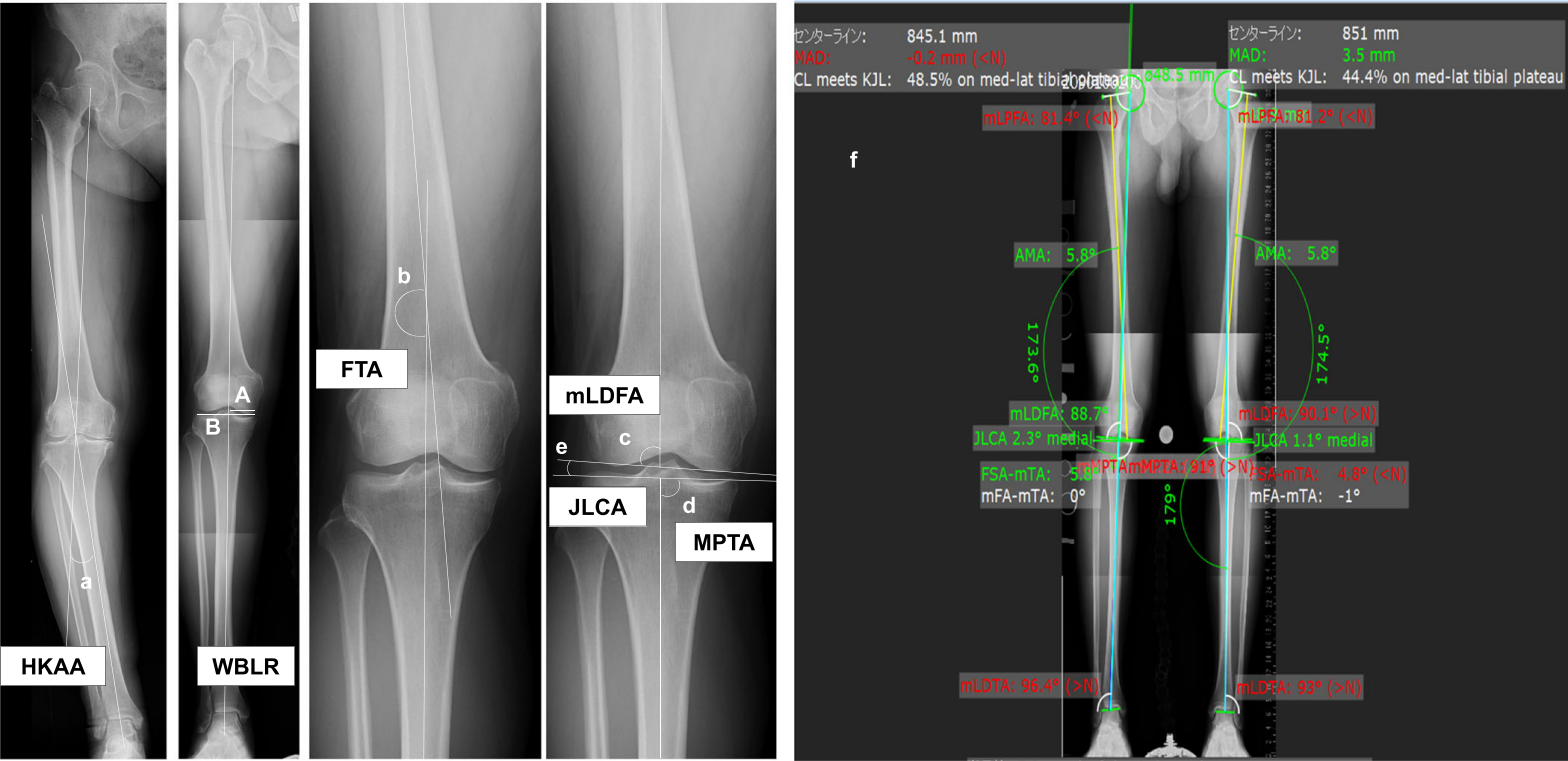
Radiographic parameters in lower limb alignment. HKAA, hip–knee–ankle angle (a), FTA, femorotibial angle (b), mLDFA, mechanical lateral distal femoral angle (c), MPTA, medial proximal tibial angle (d), JLCA, joint line convergence angle (e), WBLR, weight‐bearing line ratio. *The weight‐bearing line ratio was calculated as A/B. (f) Example of measurement using mediCAD.
